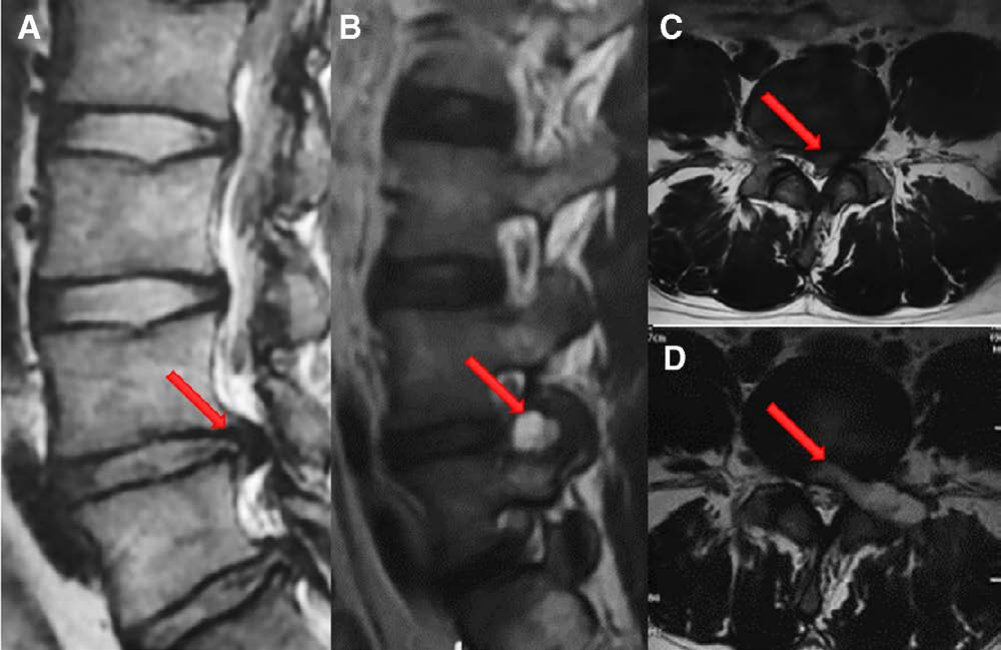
MRI images of Case 1 at the first and second admissions. (A, C) MRI of case 1 on the first admission showed that the L4/5 intervertebral disc herniated posteriorly, compressing the dural sac. (A) T2-weighted sagittal image. (C) T2-weighted cross-sectional image. (B, D) MRI of case 1 on the second admission showed an enhanced signal in T2-weighted images at the L4/5 level. (B) T2-weighted sagittal image. (D) T2-weighted cross-sectional image.
